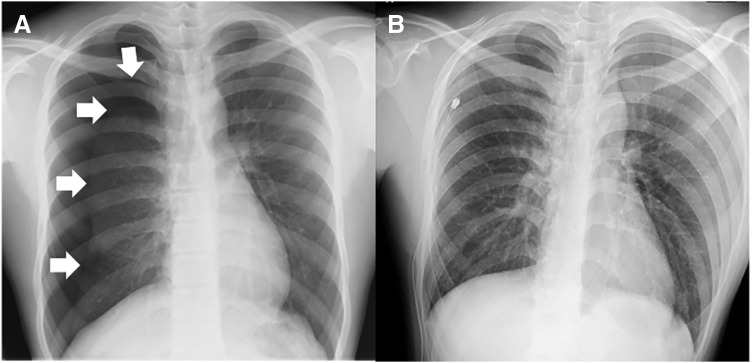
X-rays before (A) and after (B) insertion of the thoracic drain. (A) Chest radiograph showing a right pneumothorax (arrows). (B) After insertion of an 18-Fr trocar catheter. The right lung had expanded well.
